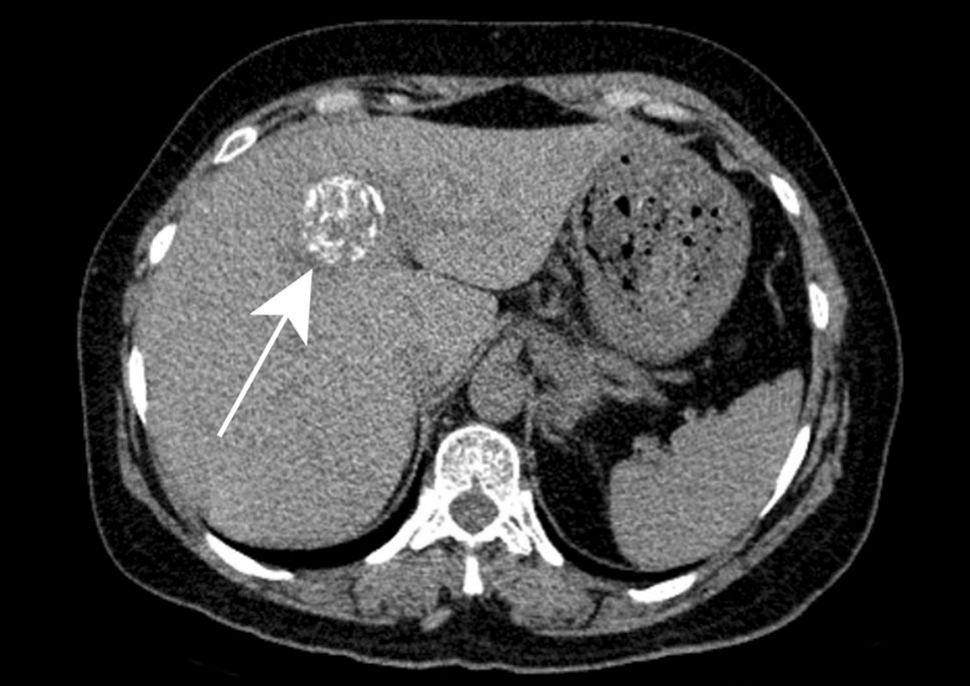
Cystic echinococcosis type CE5 in a 50-year-old female patient with an incidental solid mass reported on ultrasound. Axial precontrast CT shows a round highly calcified lesion in segment IV (white arrow)
